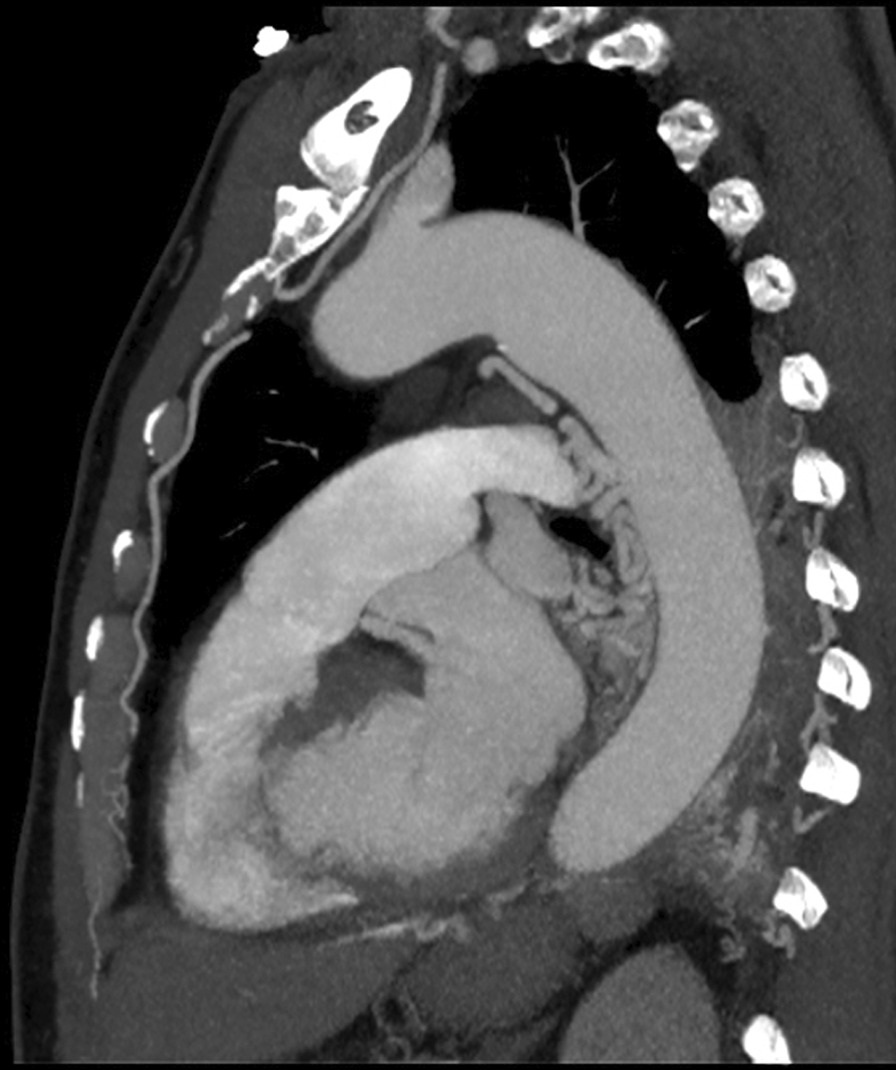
Computed tomography angiophy of bronchial artery (sagittal plane): tortuous and dilated branches of left bronchial artery
